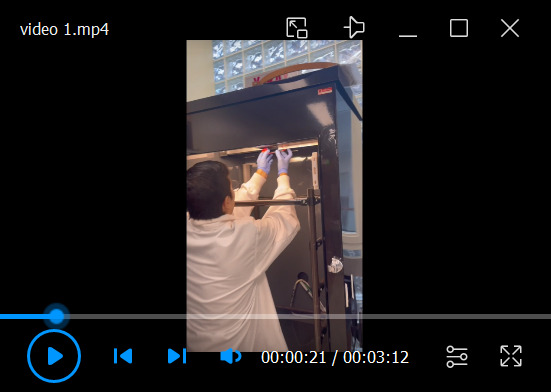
Video tutorial showing the sample preparation and X-ray imaging procedure
